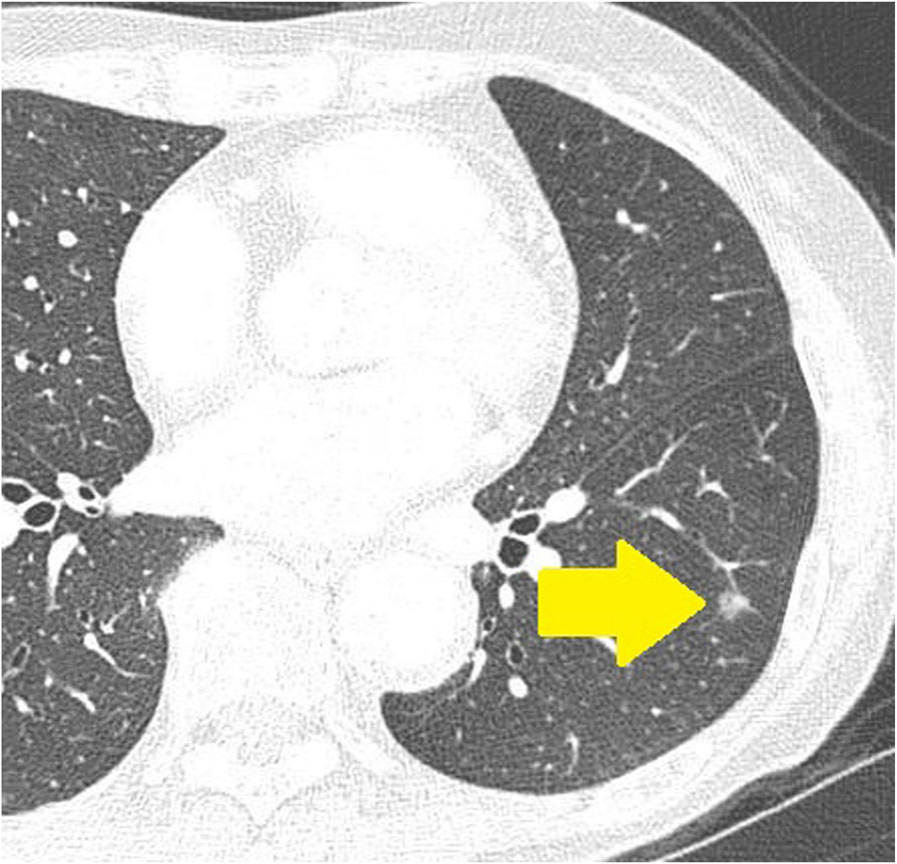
Chest computed tomography. a 6-mm left lower lobe nodule with ground glass opacity
